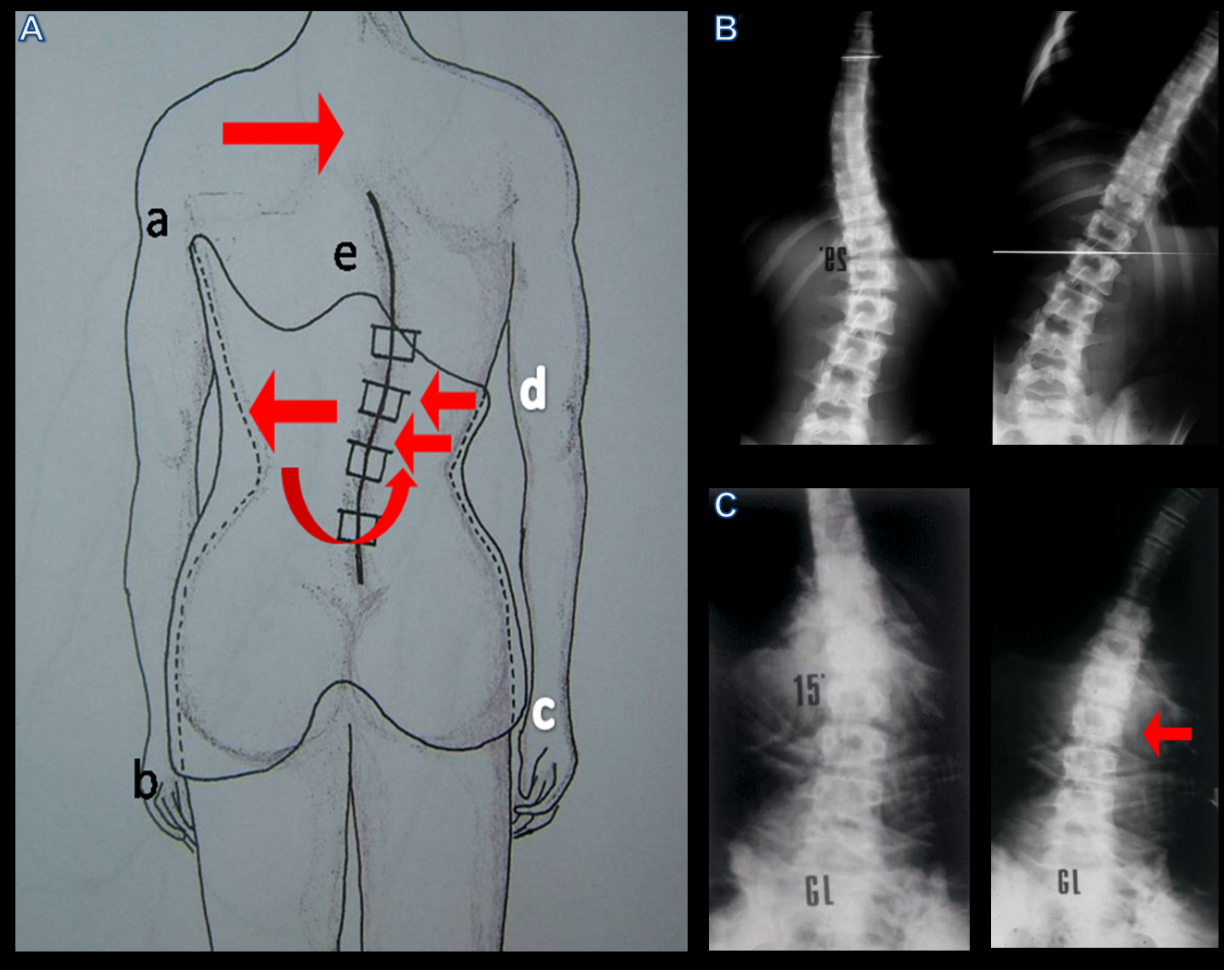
Dynamics bound by the geometry of the brace. On the convex side, the superior margin ends under the apex vertebra, allowing the lateral flection movement to induce the deflection of the curve and the realignment of the spine under the apex vertebra. The posterior edge is modelled with a central active prominence which is uncomfortable and compels the patient to perform an anterior translation that allows the hyperlordosis correction (A). Such a profile imposes a particular dynamics, for which the anterior flection movements can be performed only together with a lateral flection and a rotation in the direction of the correction of the curve. The bending X-Ray exam executed on the convexity side without (b) and with the brace (c) confirms the theoretical presuppositions showing the remarkable behaviour diversity between the dynamics of the free and the bound spine.
